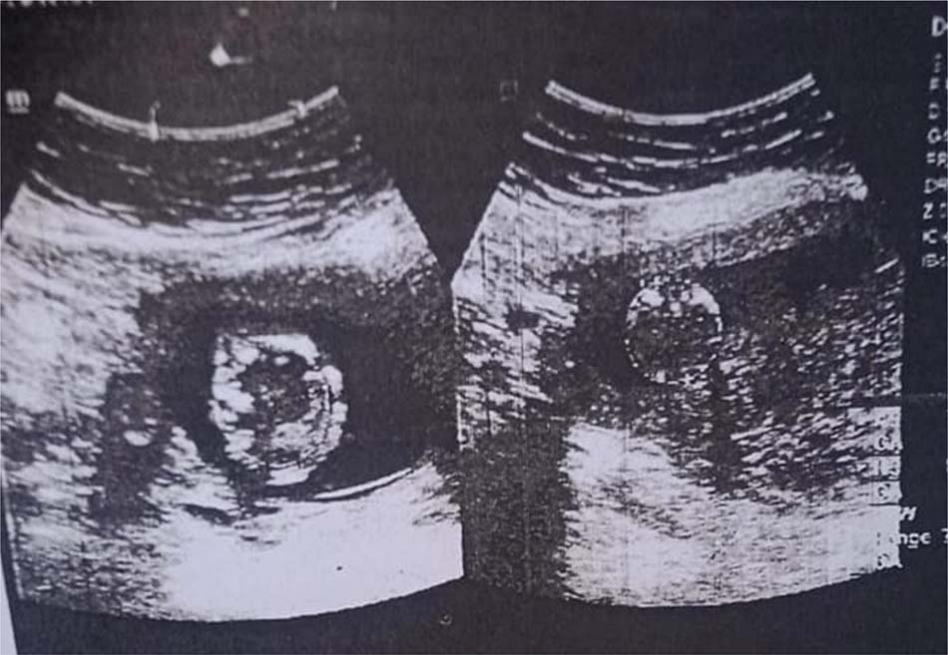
Ultrasound confirmation of pregnancy.
